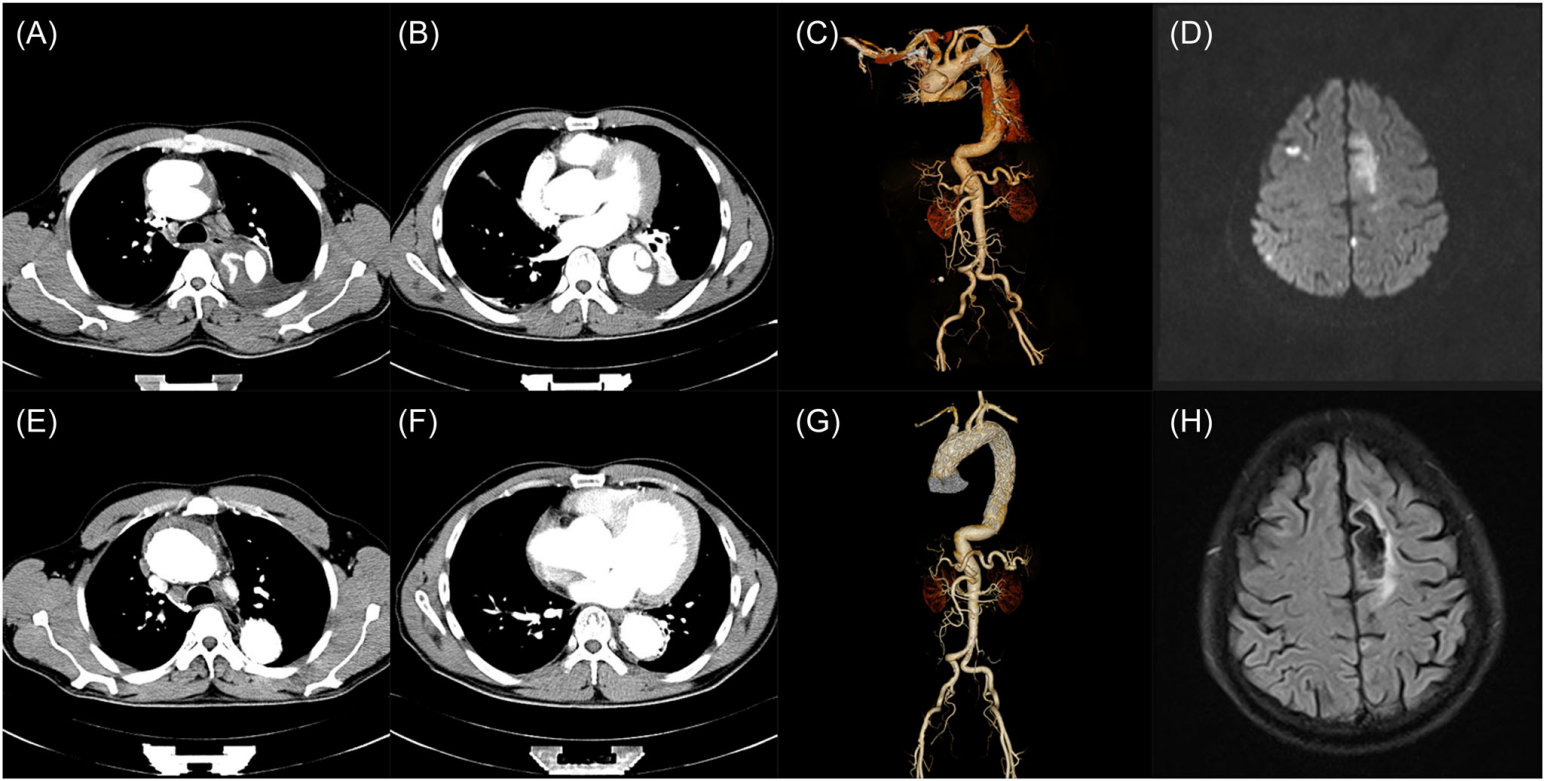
(A) CTA showed a torn diaphragm from the ascending aorta to the left ventricle. (B) Another small laceration at the arch descent, and a false lumen were observed. (C) Three‐dimensional reconstruction CT showed both lesions simultaneously. (d) Postoperative DWI‐MRI of the head suggested multiple acute cerebral infarctions in the brain parenchyma. (E–G) Thoracic aortic CTA 1 year after the operation showed that the dissection flap ended proximally, and the false lumen was obliterated. (H) T_2_‐FLAIR MRI of the head showed that the infarcts had softened. CTA, Computed tomography angiography.
